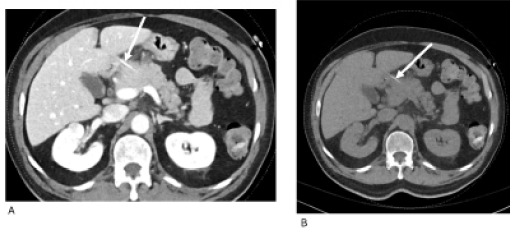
Axial computed tomography, with contrast (A), and without contrast (B), showing hyperdense linear foreign body (arrow) centered between the pancreatic head and antrum/duodenal region with adjacent inflammation.
